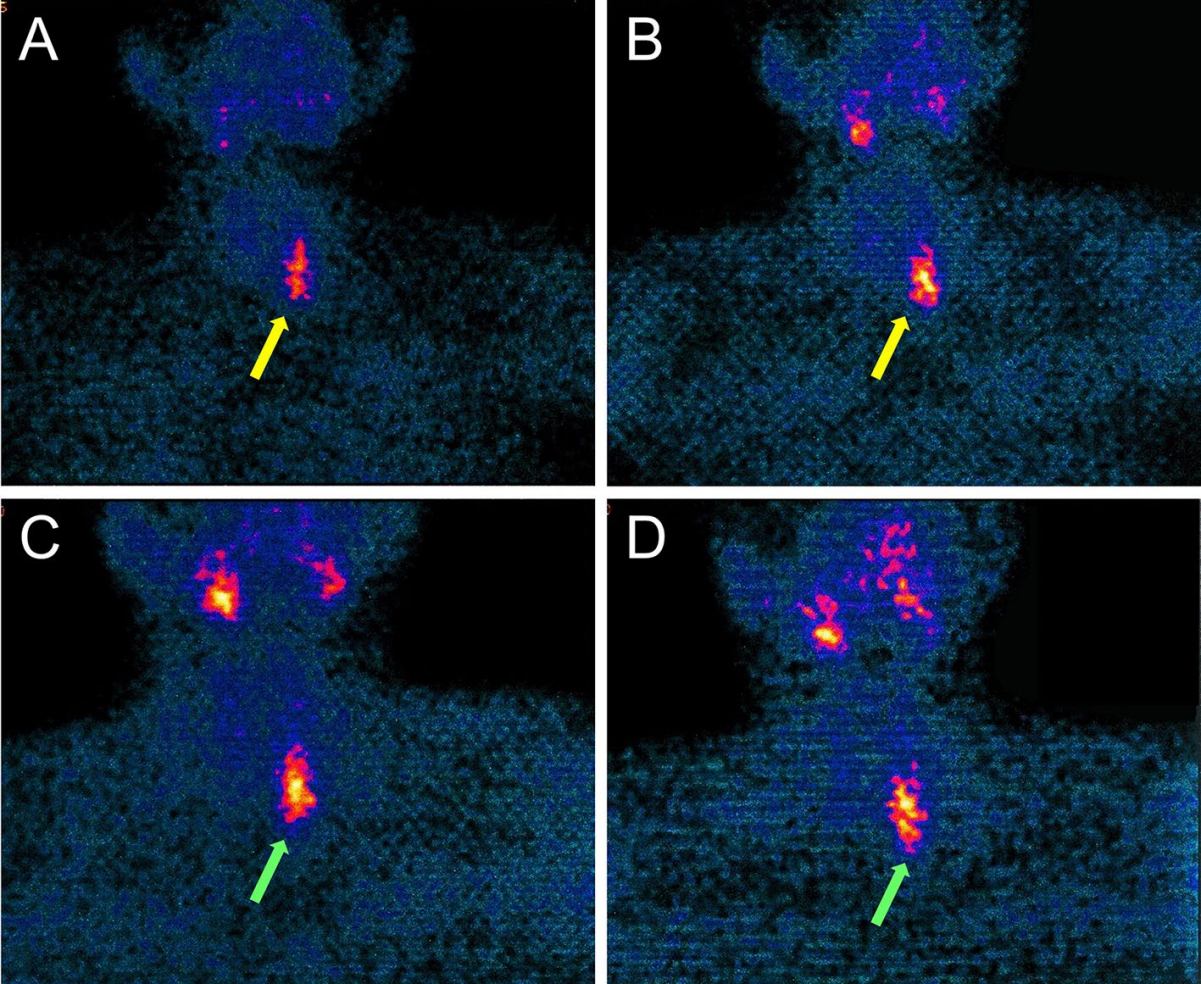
Early (A, B) and late (C, D) parathyroid SPECT imaging with [^99m^Tc]Tc-sestamibi suggesting the presence of hyperfunctioning parathyroid tissue in the lower left parathyroid lodge (yellow and green arrows)
